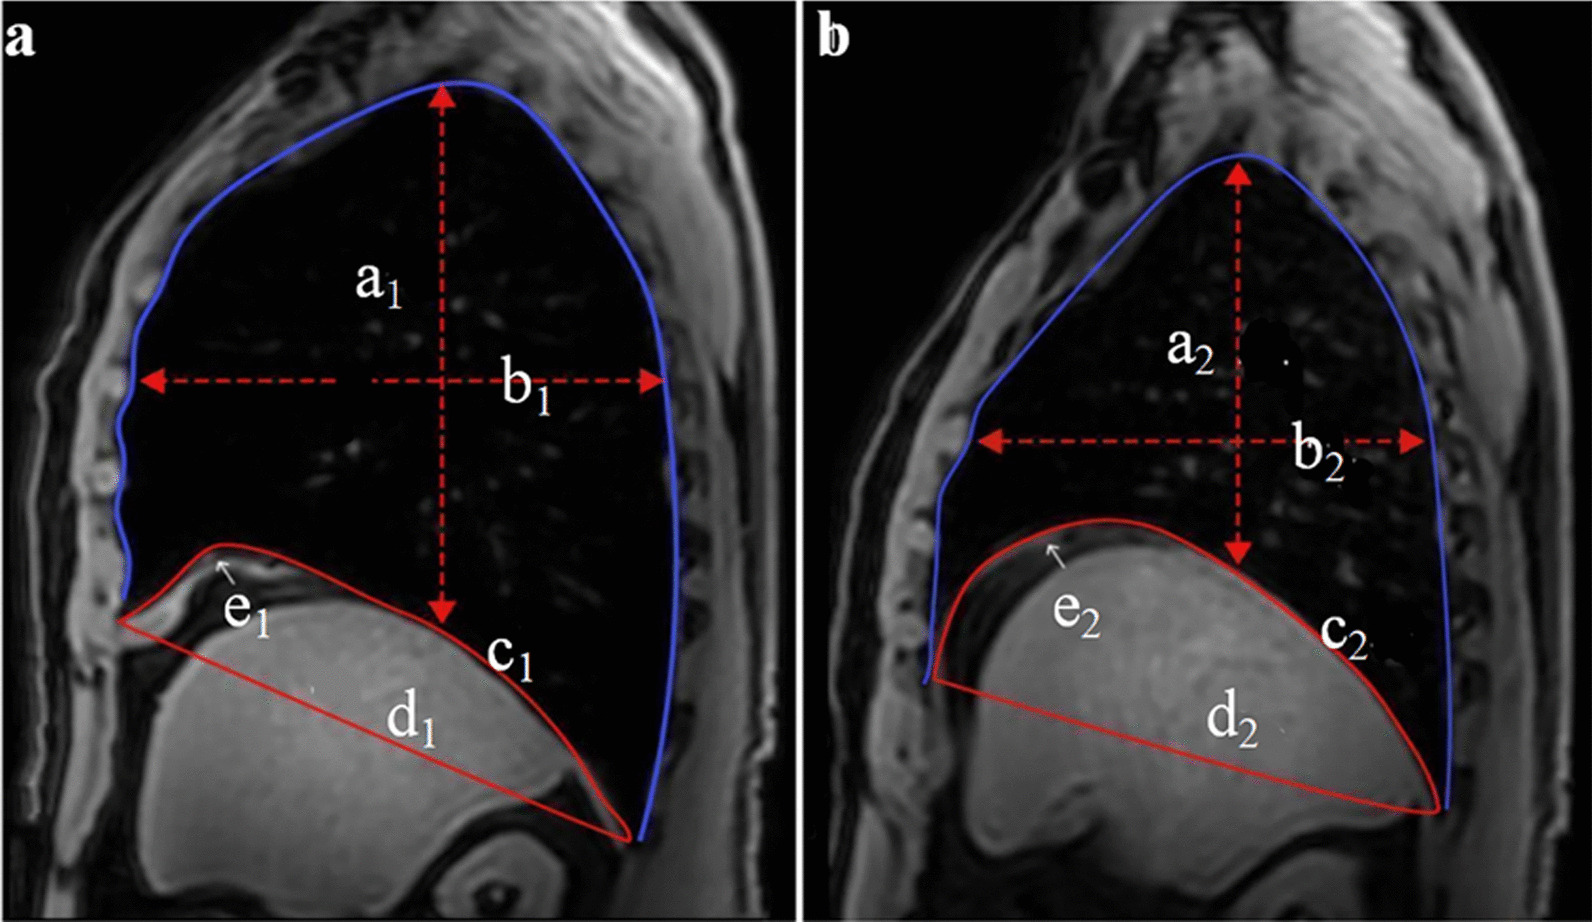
Methods for measuring diaphragm function parameters at the end of maximal breathing. The image shows a single frame of the right sagittal magnetic resonance imaging data for one participant. The lung area, which is the area inside the lung contour (coloured blue) at the end of inspiration (a) and expiration (b). a_1_, a_2_ present the upper-lower diameters, which was the vertical distance between the lung apex and hemidiaphragm is measured at end of inspiration (a_1_) and expiration (a_2_), and the two dimensions are subtracted to obtain the diaphragmatic displacement by length (a_1_–a_2_). b_1_, b_2_ present the anterior–posterior diameter of the chest, which was the trans-vertical anteroposterior dimension of the upper and lower thoraces at the right mid-sagittal planes measured at end of expiration (b_2_) and inspiration (b_1_), and the two dimensions are subtracted to obtain the chest wall motion (b_1_–b_2_). The white arrow shows the diaphragm thickness (DT) at the end of maximal inspiration (e_1_) and expiration (e_2_). c_1_ presents the diaphragm length, which is the length across the diaphragmatic dome from the anterior to the posterior chest wall margin at the end of maximal inspiration. c_2_ presents it at the end of maximal expiration; d1 presents the linear distance of the anteroposterior costophrenic angle at the end of maximal inspiration; d_2_ presents it at the end of maximal expiration. Diaphragmatic dome factor (ins) = c_1_/d_1_. Diaphragmatic dome factor (exp) = c_2_/d_2_. Diaphragm thickening fraction (DTF) = (DT at end of maximal inspiration) – (DT at end of maximal expiration)]/ (DT at end of maximal expiration) × 100%
